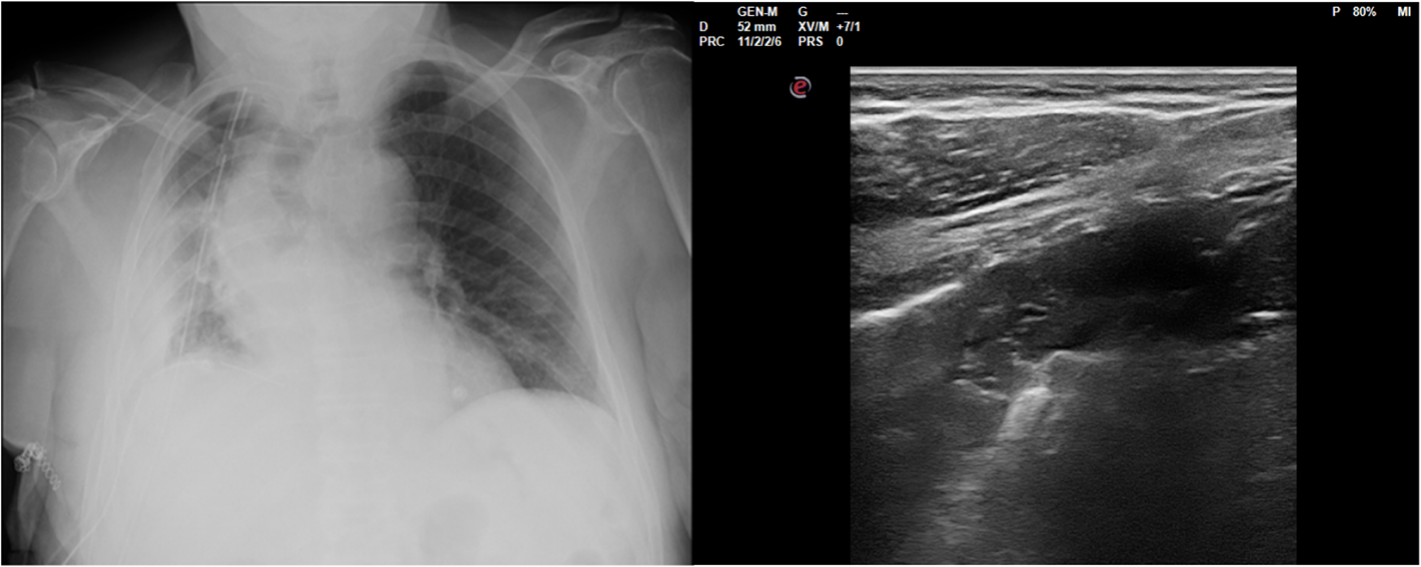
The same case of Fig. 2. Chest US reported LC compatible with obstructive atelectasis and reduction of volume of the hemithorax. Chest X-ray was required for a panoramic view
